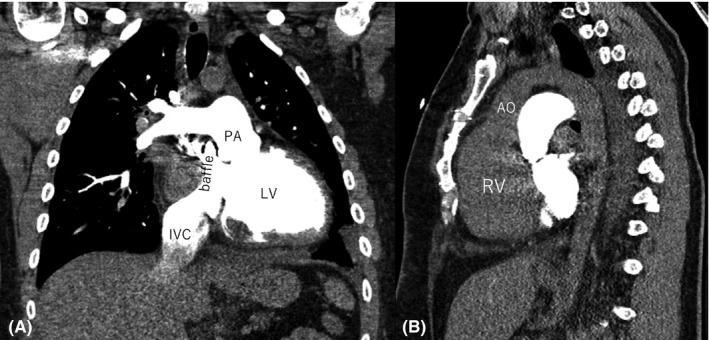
Preoperative enhanced computed tomography. A, Frontal view demonstrates that blood flow of SVC and IVC are redirected through the baffle toward the mitral valve and the LV connected with PA. B, Sagittal view shows that the systemic RV and aorta are located anteriorly with respect to the pulmonary ventricle and the pulmonary trunk. PA, pulmonary artery; LV, left ventricle; IVC, inferior vena cava; SVC, superior vena cava; RV, right ventricle
